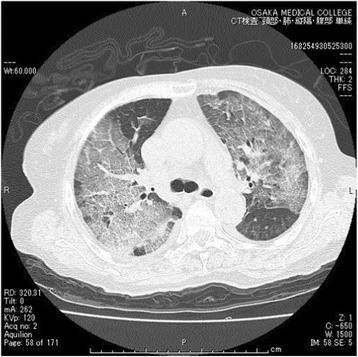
Computed tomography indicated extensive bilateral infiltrates
